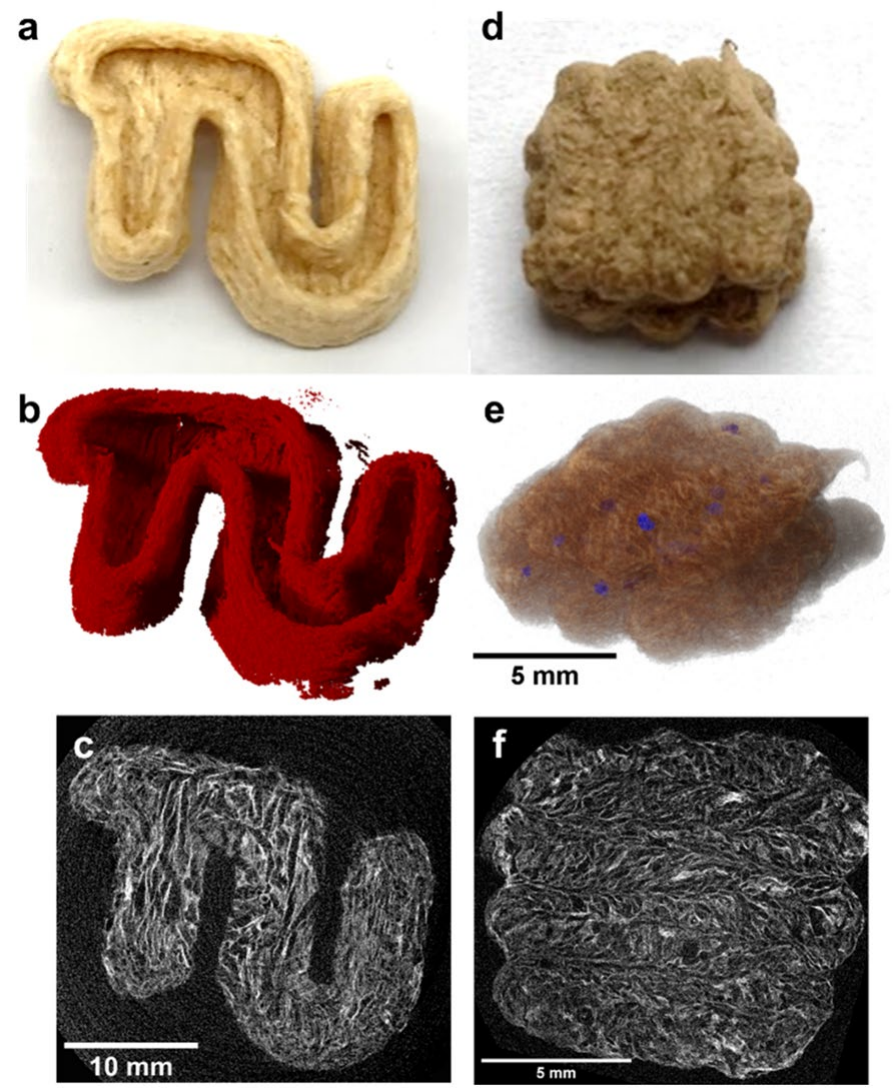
a Photographic image of a printed "TU" logo, b 3D image of the reconstructed volume, c µ-CT tomographic image of a slice through its bottom layer, d photographic image of the printed bulk sample, e 3D oblique view of the printed bulk sample with enclosed air bubbles in blue and f µ-CT tomographic image of a slice through the middle layer of a bulk sample acquired with higher resolution
